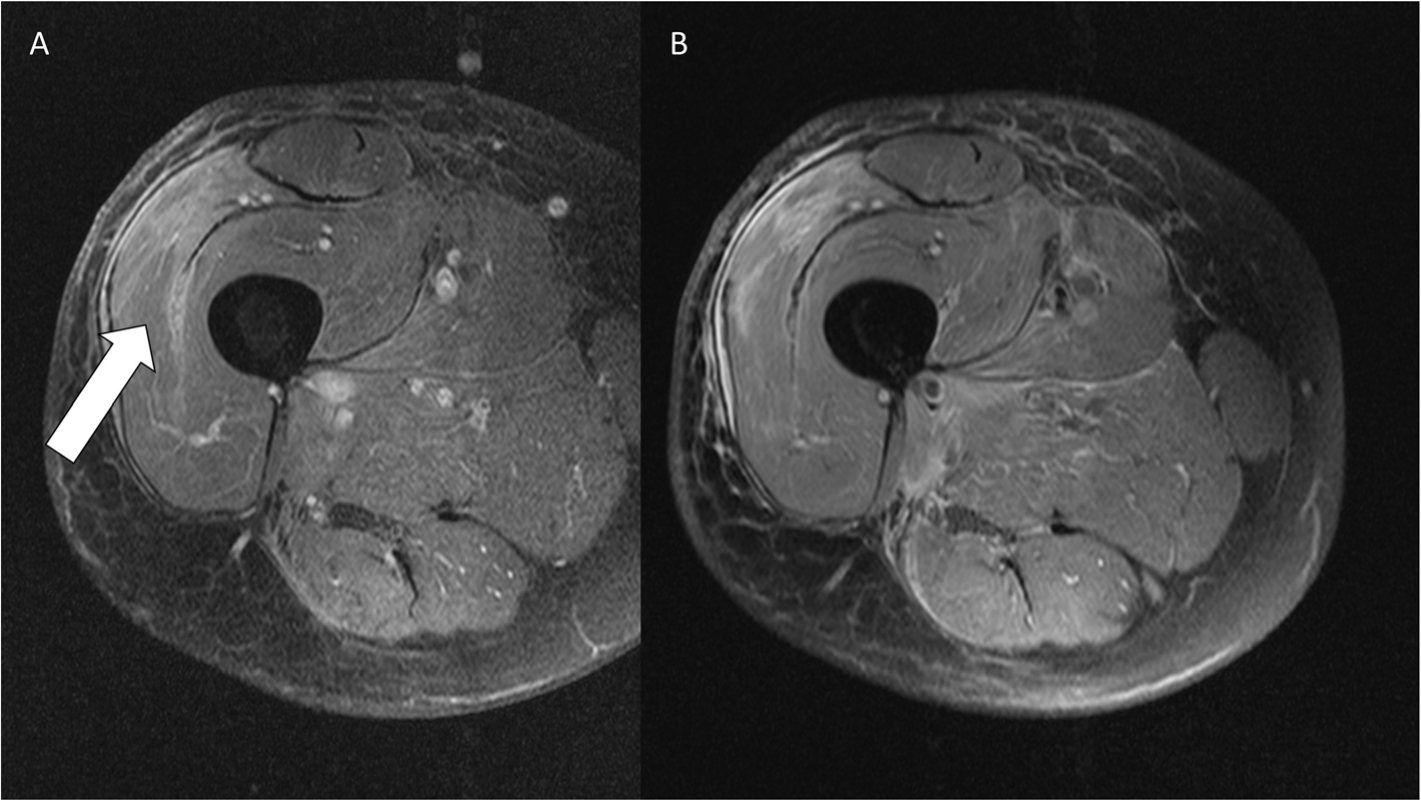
1.5-T MRI of the thigh (a: contrast-enhanced T1-weighted image with fat saturation in axial view; b: Proton density-weighted image with fat saturation in axial view). Post-treatment muscle edema (white arrow) is shown without recurrent STS
